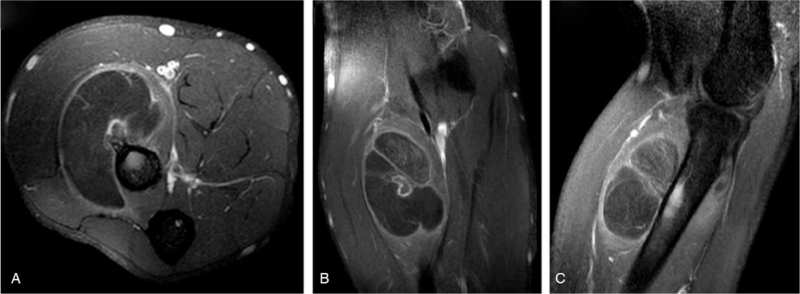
Parosteal lipoma of the proximal anterolateral forearm. Axial fat saturated T1-weighted (A), coronal fat saturated T1-weighted (B), and sagittal fat saturated T1-weighted (C) MRI of the right elbow and forearm after contrast administration showed enhancement of the septae and periosteum within the marginally enhanced parosteal lipoma.
